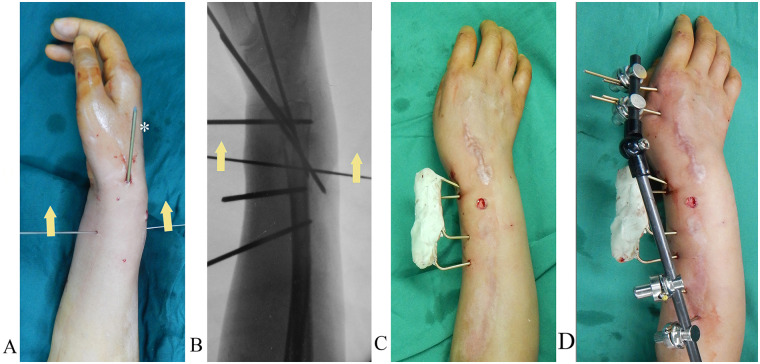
(A) A 2.5 mm pin was inserted manually, 5–10 mm distal to the fracture site (avoiding late skin laceration during distraction), and slid into the fracture site. The pin was continuously passed through the fracture site and it exerted pressure on the dorsal skin incision. By pulling both ends of the pin distally (arrows), the distal bone fragment was distracted to restore the normal length of the radius and meanwhile to restore volar tilt and radial inclination. To maintain the reduction, one or two 3.0 mm pins (*) were provisionally introduced, obliquely in a distal-to-proximal direction. (B) A bony distraction maneuver on X-ray. (C) Two to three 3.0 mm pins were inserted in the distal fragment and two pins in the proximal fragment. The pin ends were bent toward the fracture site about 2 cm away from the skin. Bone cement was mounted on the pin ends. (D) A bridging external fixator was applied against axial load.
